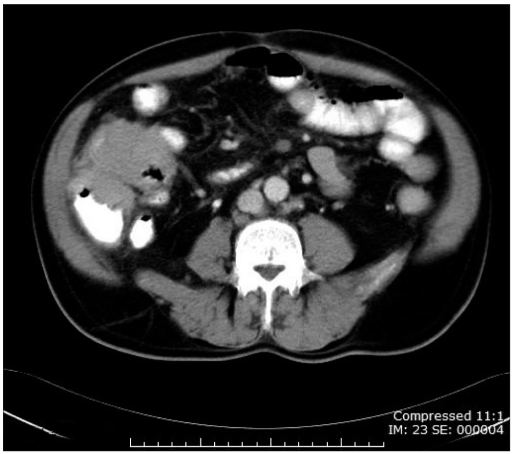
CT scan demonstrating a cecal mass
